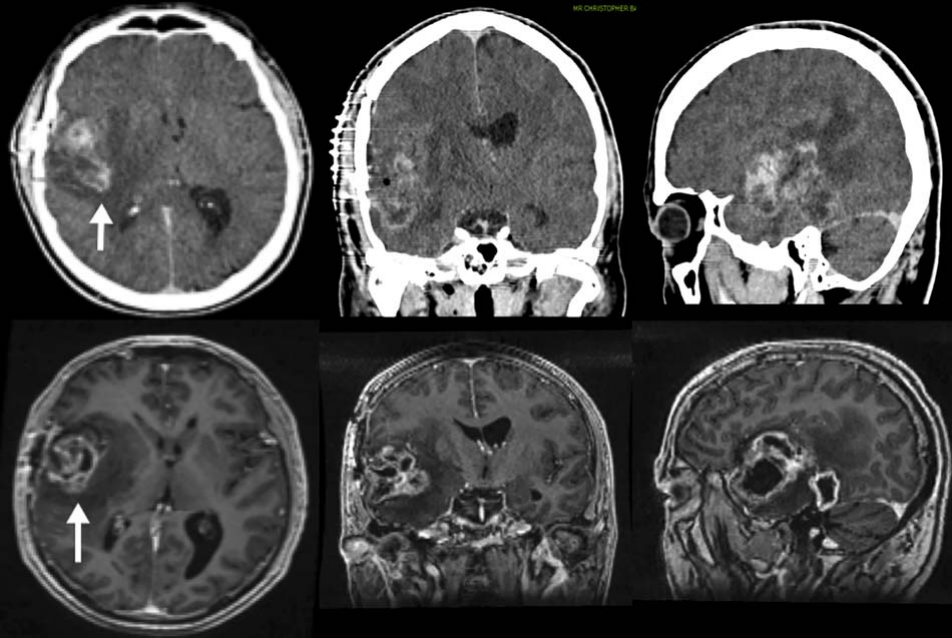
(A–C) (Top Row: axial, coronal and sagittal) contrast CT conducted < 72 h post-operatively demonstrating significant lesional reduction and mass effect (arrow). (Bottom row: axial, coronal and sagittal) Contrast T1W MRI demonstrating multiple areas of lesional enhancement representing a STR but a slight reduction in oedema (arrow). Patient could not tolerate the usual post-operative MRI due to poor neurological status. This was conducted at 14 days.
